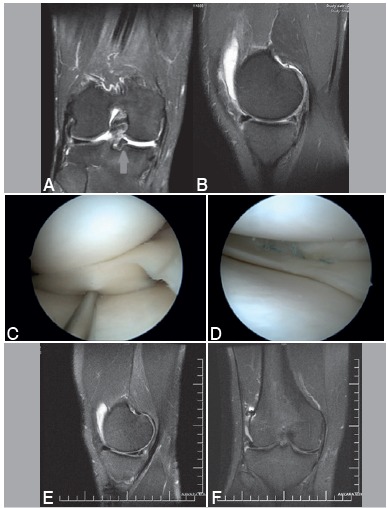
A displaced BHMT associated with ACL tear seen on the coronal (A) and sagittal image (B) of MRI. The displaced meniscal part migrated to the intercondylar notch and remarked with an arrow. The displaced tear was seen on arthroscopy (C). Posterior meniscal tear was sutured with the all-inside technique with 2 sutures. The middle part of the tear was sutured with the inside-out technique (D). The repaired meniscus was almost completely healed at the postoperative 26-month evaluation on sagittal and coronal images of MRI (E, F).
